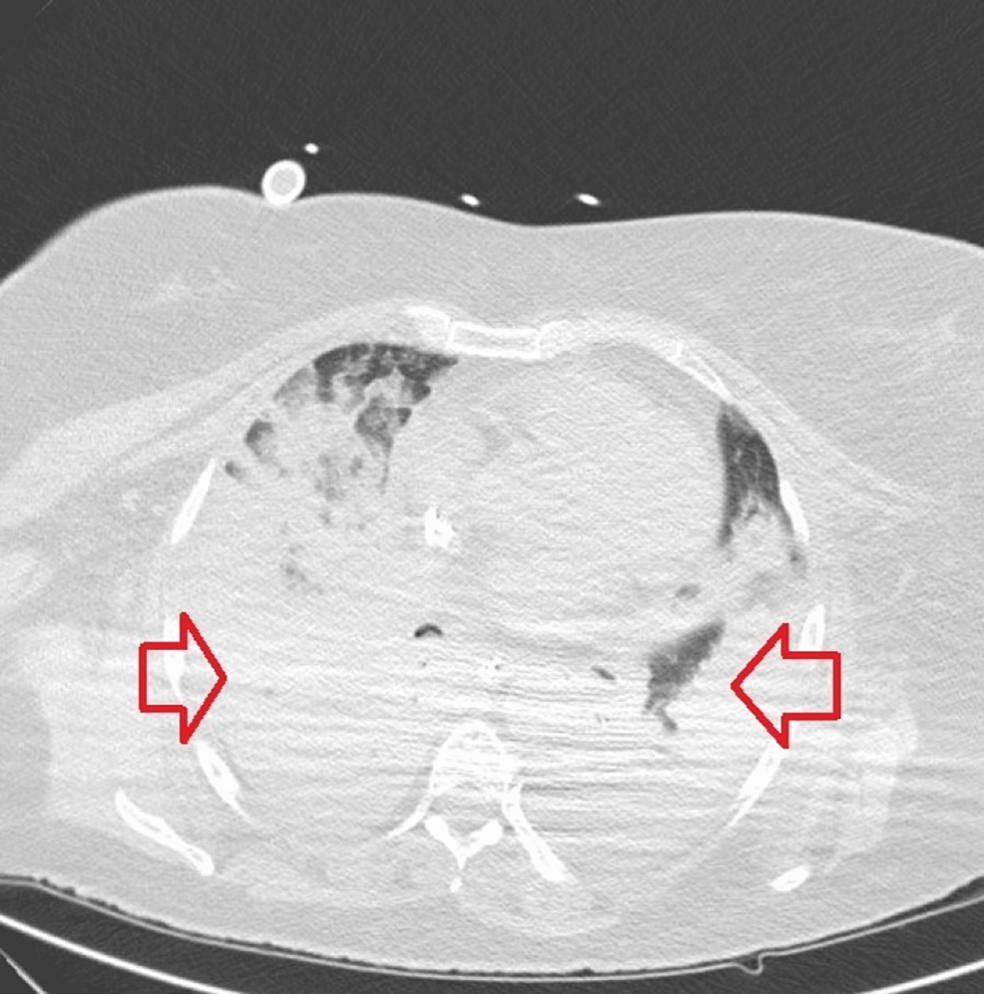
Thoracic CT scan (axial view) on the first day of hospitalization The red arrows show consolidation under venovenous extracorporeal membrane oxygenation
